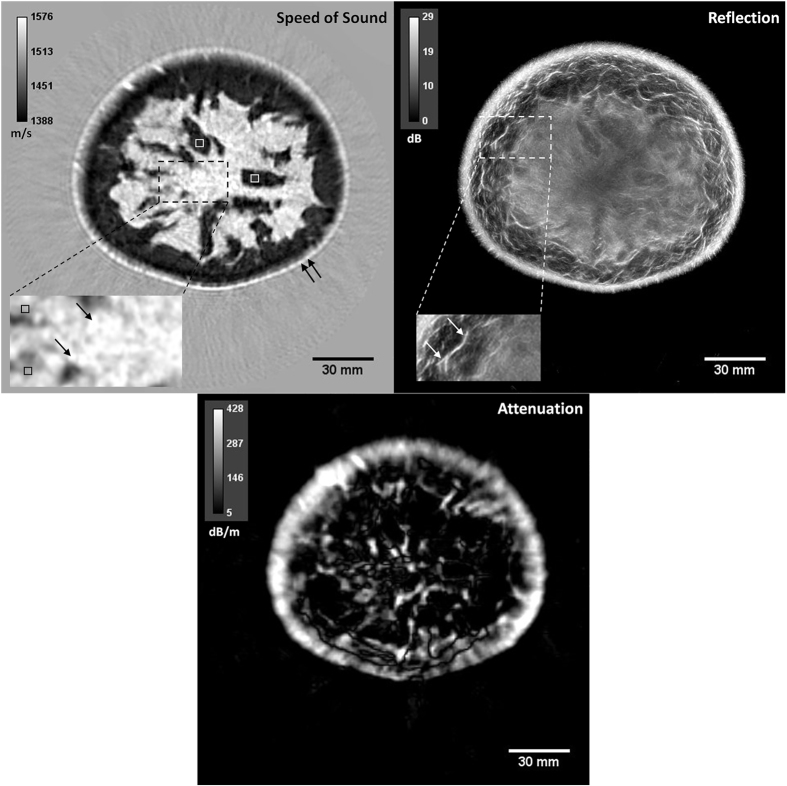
Representative multimodality QT ultrasound images of a volunteer’s breast. Left: speed of sound, middle: attenuation, and right: reflection images. Example ROIs are identified within the images. Speed of sound image: The white and black squares in the speed of sound image mark fat and glandular tissue, respectively. Single and double black arrows mark ductal tissue and skin, respectively. Reflection image: Single white arrows mark the connective tissue identified as Cooper’s ligaments.
